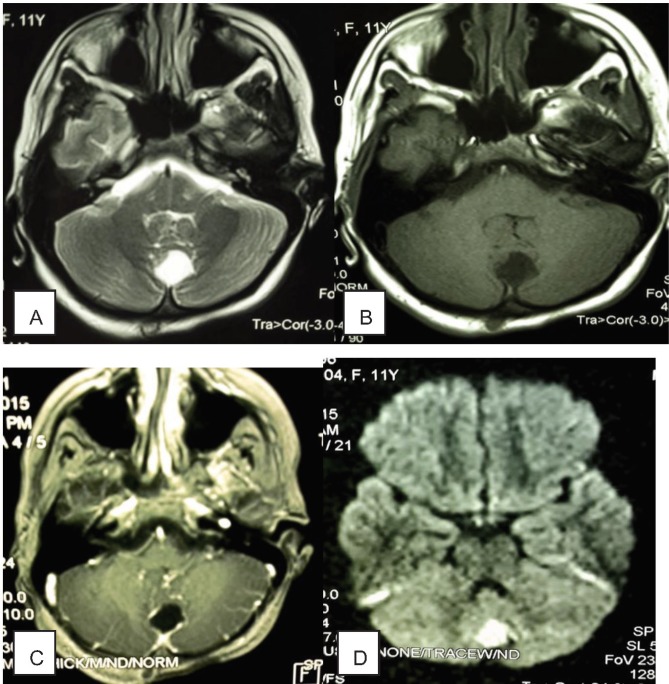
A. Axial T2 Weighted brain MRI and B. Axial T1weighted brain MRI shows an extra-axial CSF density lesion in posterior mid aspect of posterior fossa. C. Axial T1 Weighted contrast enhanced brain MRI reveals rim enhancement of the lesion. D. DWI (Diffusion Weighted Imaging)sequence demonstrates restriction in the lesion.
